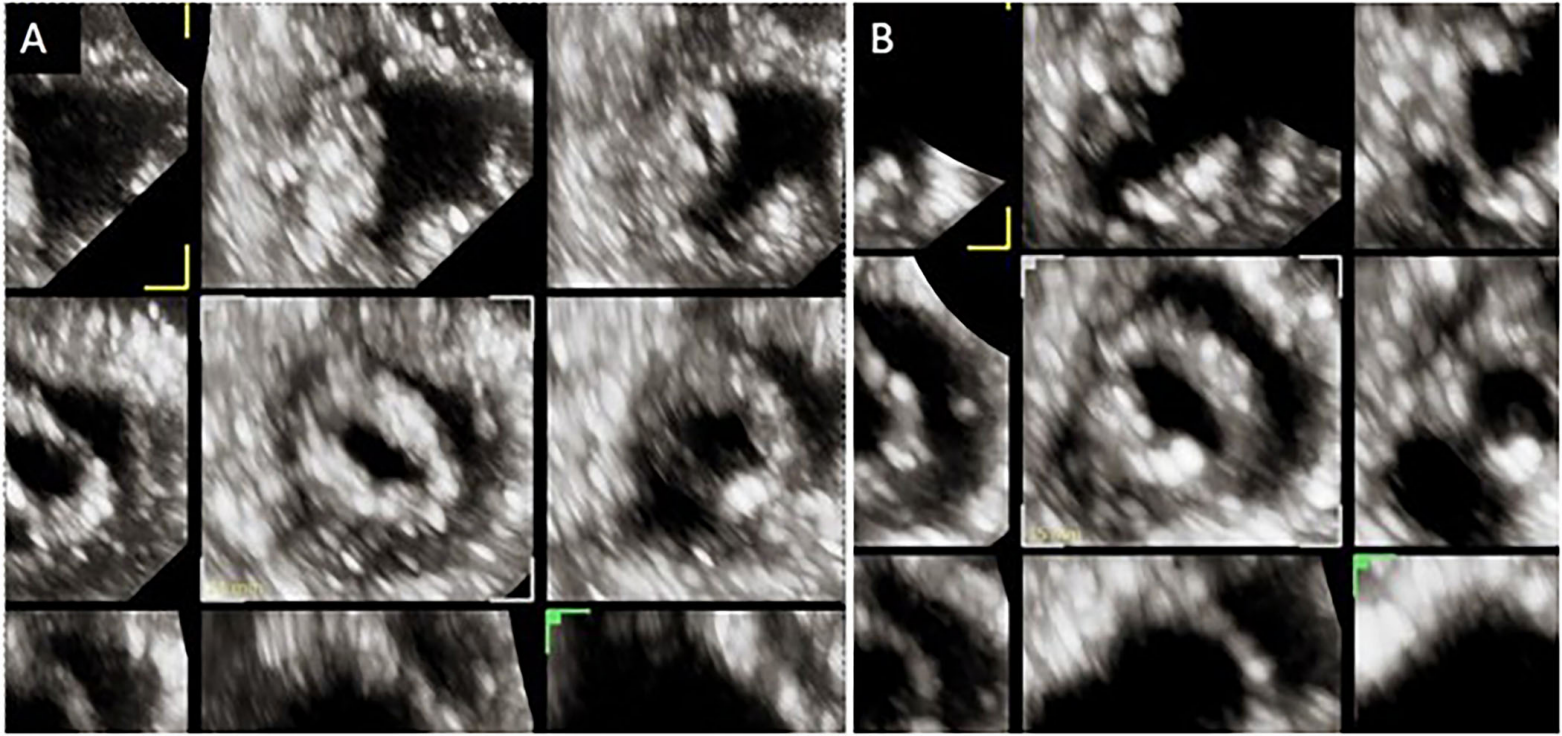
Transthoracic echocardiography—short‐axis view of the mitral valve after the initial Inque balloon inflation (A) and lithotripsy‐assisted balloon inflation (B). [Color figure can be viewed at wileyonlinelibrary.com]
